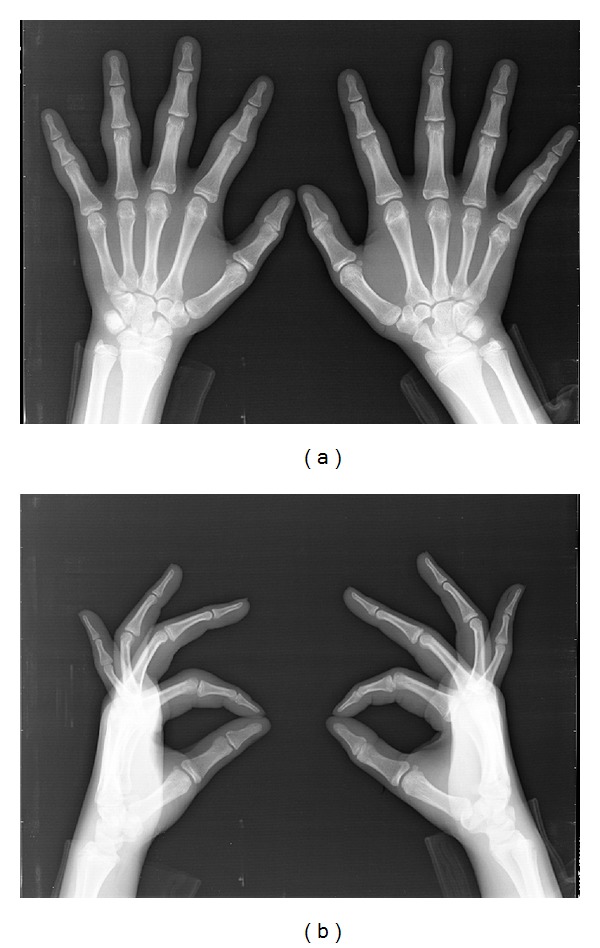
Plain radiograph of bilateral hands at the first examination. No bony erosive and joint destruction around the PIP joints on anteroposterior (a) and lateral (b) images.
